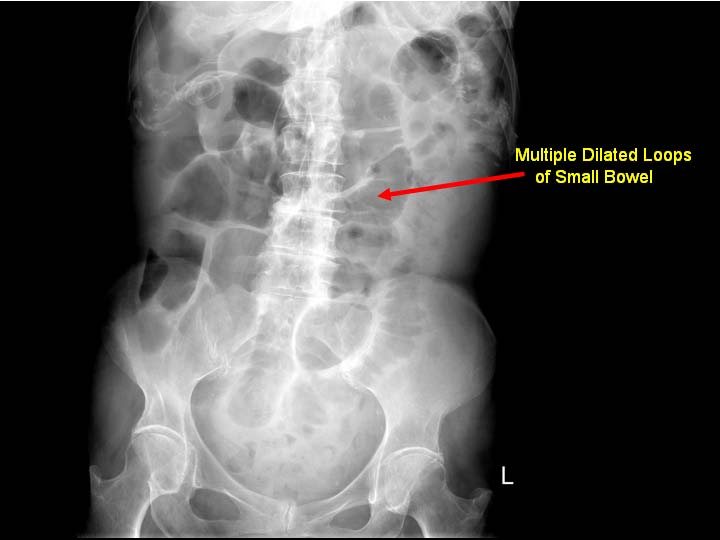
Abdominal X-ray displayed multiple dilated loops of small bowel.
